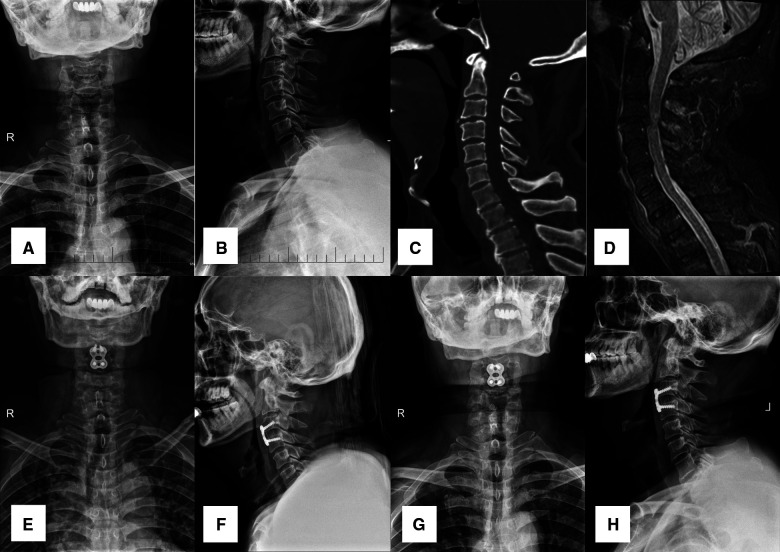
A 56-year-old male patient diagnosed with TCCS. (A,B) preoperative A-P and lateral X-ray images showing the slight degenerative change of cervical spine with relative normal curvature, and slight anterior osteophyte formation. (C) preoperative CT scan presented no fracture or dislocation of the cervical spine. (D) preoperative MRI from STIR displayed significant cervical spinal cord compression at C3-4 with spinal cord signal change at the same level, while distinct prevertebral hyperintense was also detected. (E,F) postoperative A-P and lateral X-ray images at discharge indicated APCS was in the appropriate site, and disc height was restored to a normal degree. (G,H) both CA and disc height were maintained at final follow-up.
